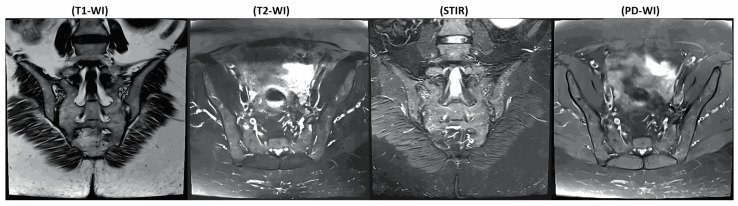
Representative T1-WI, T2-WI, STIR, and PD-WI MRI sequences of the sacroiliac joint from the same participant.
